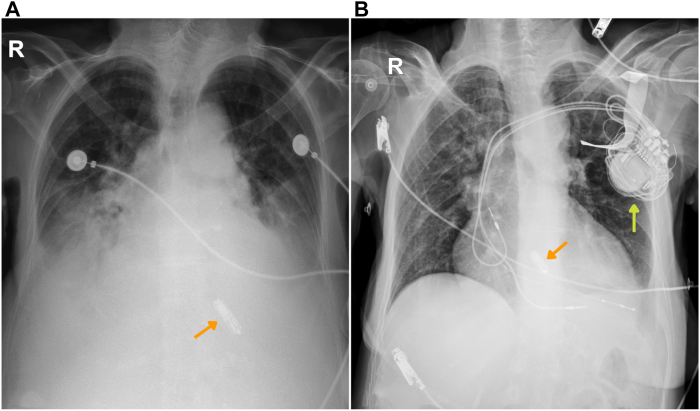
A: Chest radiograph on arrival, bilateral pleural effusion and pulmonary edema. B: Postprocedural chest radiograph showing a cardiac resynchronization therapy pacemaker device. *Orange arrows* = Micra AV device; *yellow arrow* = cardiac resynchronization therapy pacemaker device.
